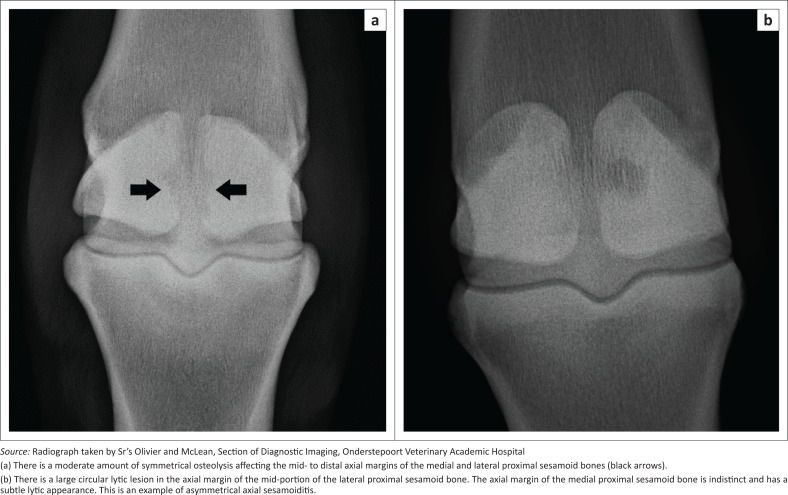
Dorsoproximal-palmarodistal oblique radiographs of the (a) left metatarsophalangeal joint of a 6-year-old Friesian stallion (b) and of the left metacarpophalangeal joint of a 15-year-old warmblood mare. Lateral is to the right of the images.
